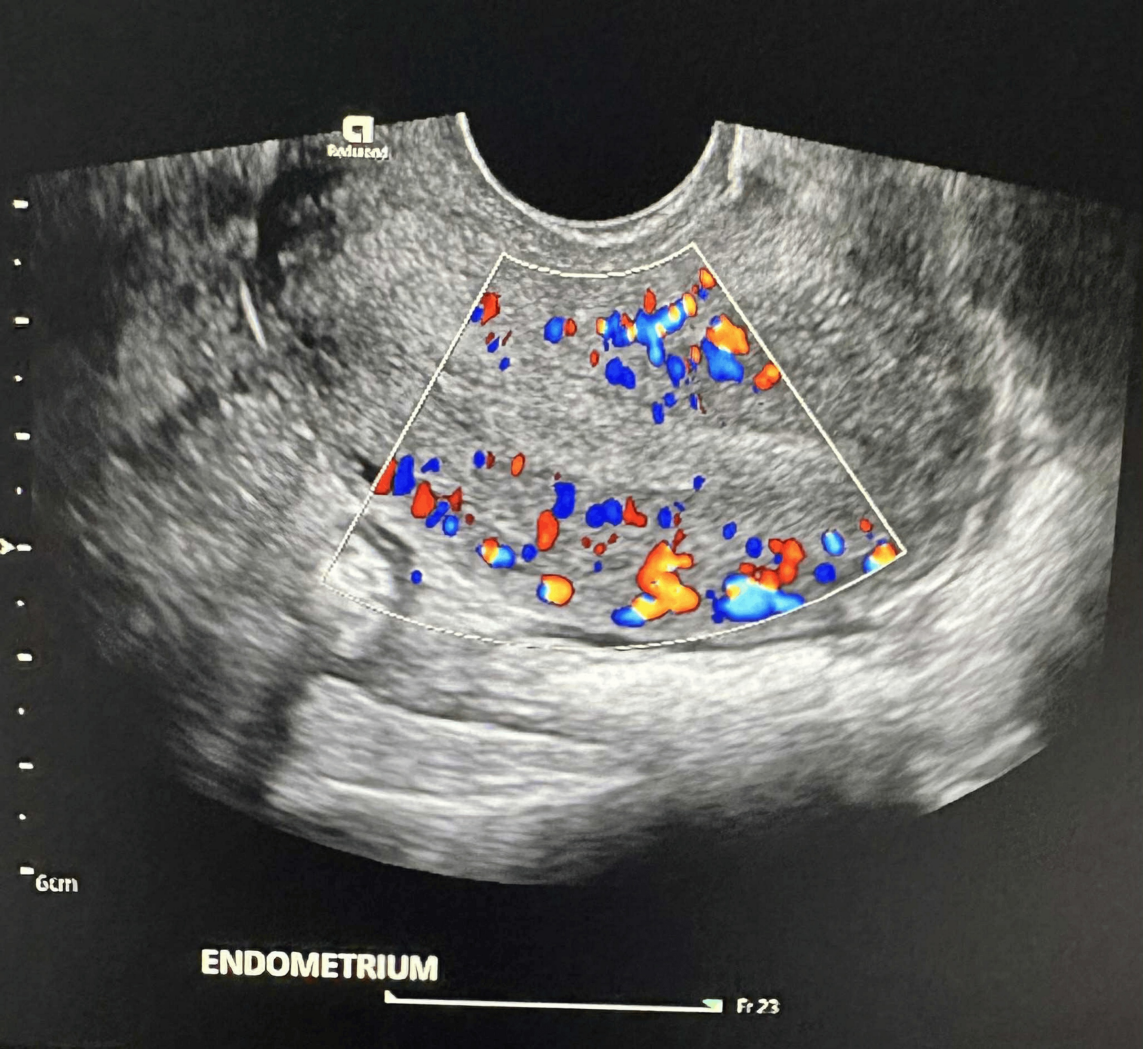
US Doppler post-miscarriage Subsequent imaging after passage of pregnancy tissue showed an empty uterus with normal endometrial lining.
